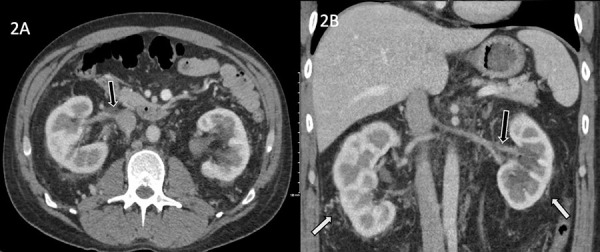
Computed tomography angiography of the kidneys demonstrating bilateral renal vein thrombosis and perinephric venous collaterals. A: Axial contrast-enhanced image demonstrates a filling defect in the distal right renal vein (black arrow) and bilateral perinephric collateral veins. There is left-sided hydronephrosis with increased density in the left renal pelvis likely representing hemorrhage. B: Coronal contrast-enhanced image demonstrates a filling defect within the proximal left renal vein (black arrow) and bilateral perinephric collateral veins (white arrow).
